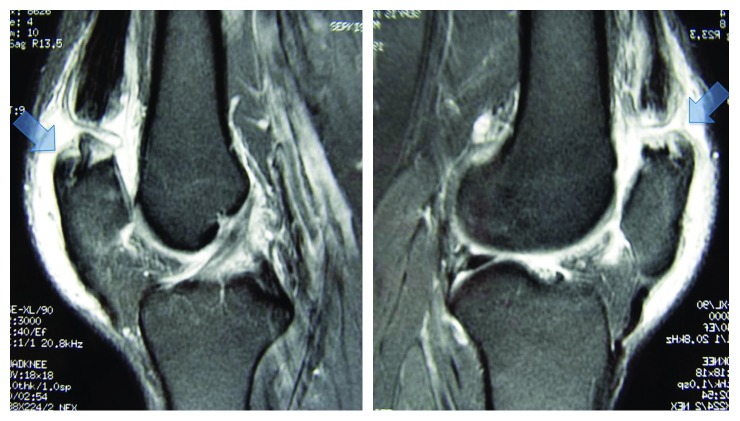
Sagittal T2-weighted MR image shows complete rupture of the quadriceps tendon with a gap between retracted quadriceps tendon and patella (right arrow). Left arrow shows the osteophyte formation at the upper end of the patella.
